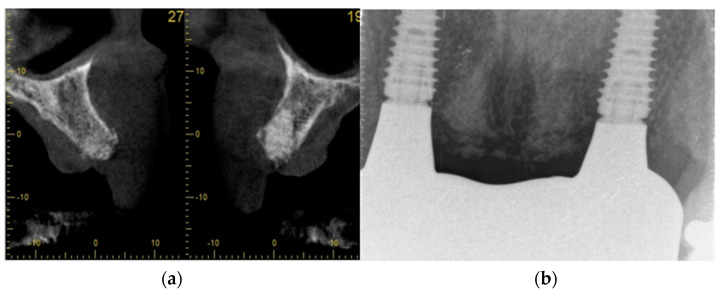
(a) shows sagittal view of a CBCT at UR2 and UL2 grafted socket sites. (b) a control periapical radiograph showing the stability of marginal bone support around the two Megagen Anyridge^®^ implants in function.
